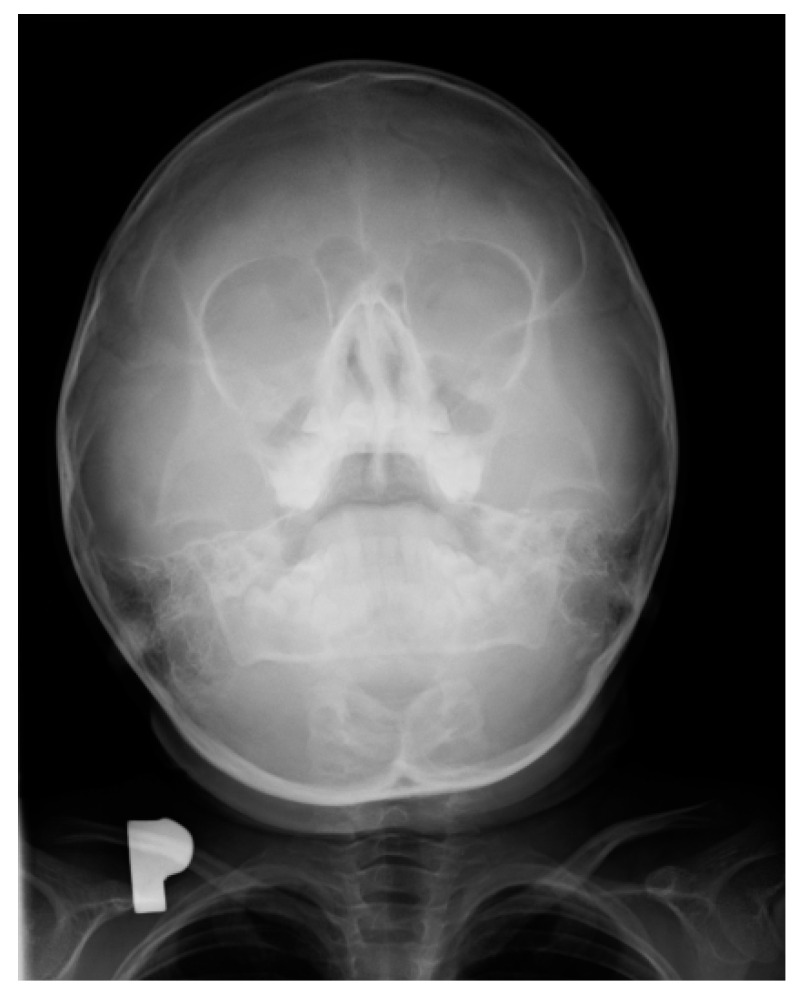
X-ray of the sinuses of a 6-year-old girl.
